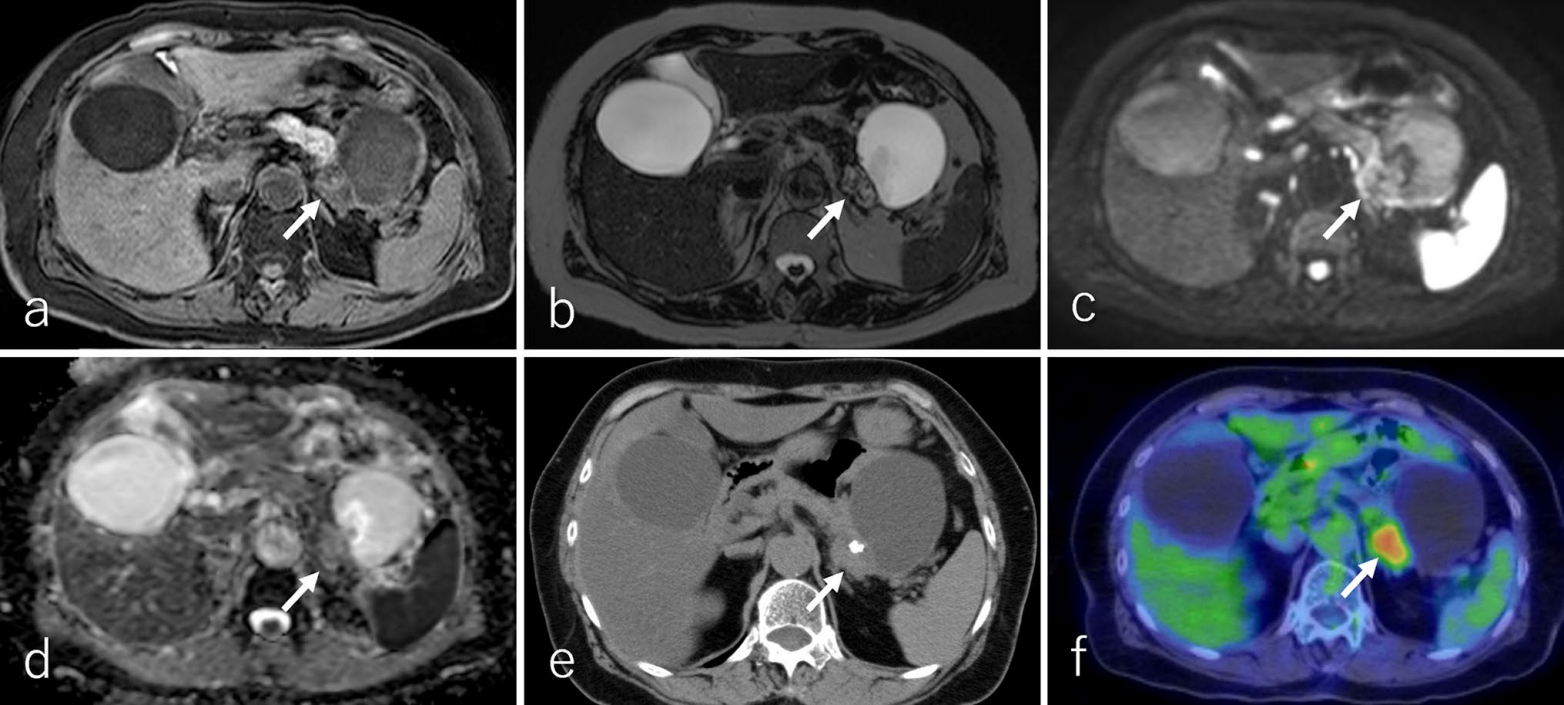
A 79-year-old female patient with colloid carcinoma (CC). a Fat-suppressed T1-weighted imaging (FS-T1WI), b T2-weighted imaging (T2WI), c diffusion-weighted imaging (DWI) with b value of 800 s/mm^2^, d apparent diffusion coefficient (ADC) map, e non-contrast-enhanced computed tomography (non-CECT) and f
